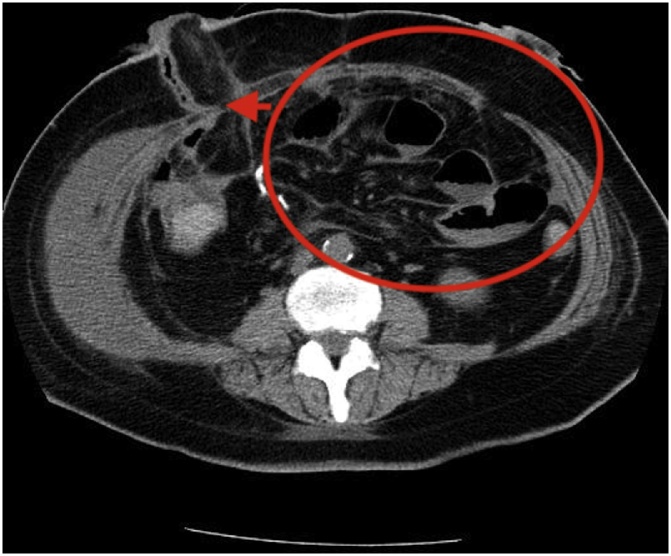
Computed tomography scan 16 days after ileostomy and colostomy.The patient complained of nausea and vomited on day 16. Computed tomography scan revealed dilation of the small bowel (circle) and angulation in the abdominal wall impinging on the ileostomy (arrow).
